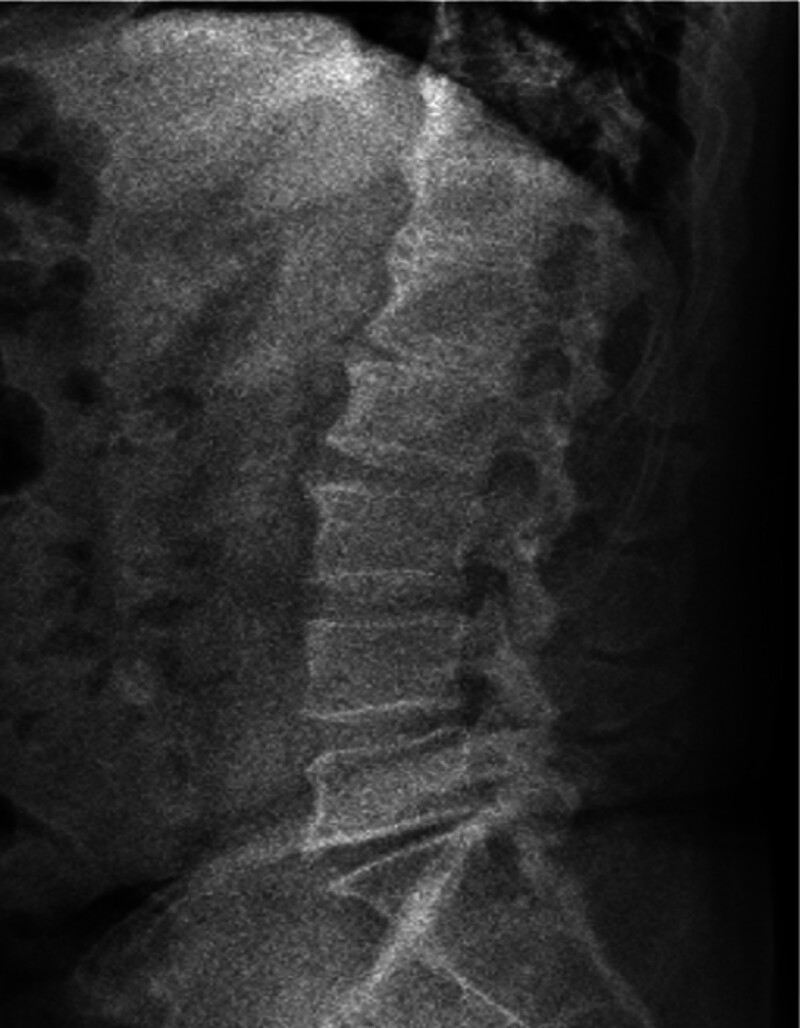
Lateral plain film of lumbar spine: it can be seen that there is osteoporosis and bone degeneration.
